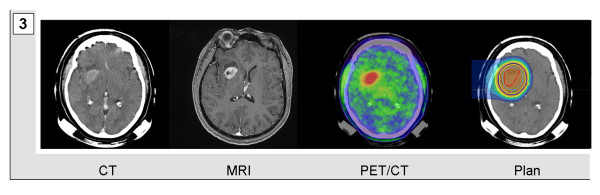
Glioblastoma multiforme in the right frontal lobe of a 48-year-old woman. Contrast-agent enhanced CT and MRI scan were fused with a FET-PET/CT examination and used to calculate a single beam carbon ion radiotherapy plan.
